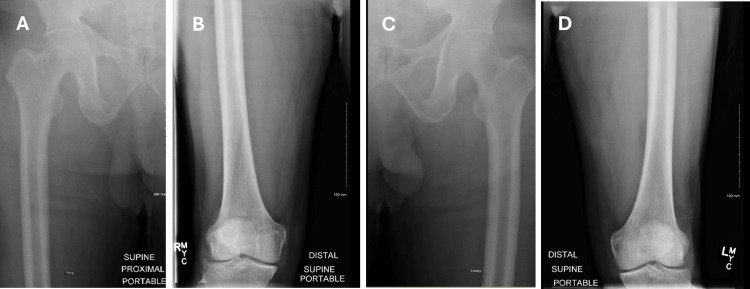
X-ray showing mild to moderate nonspecific degenerative changes of bilateral lower extremities. Images A and B correspond to the right lower extremity. Images C and D correspond to the left lower extremity
